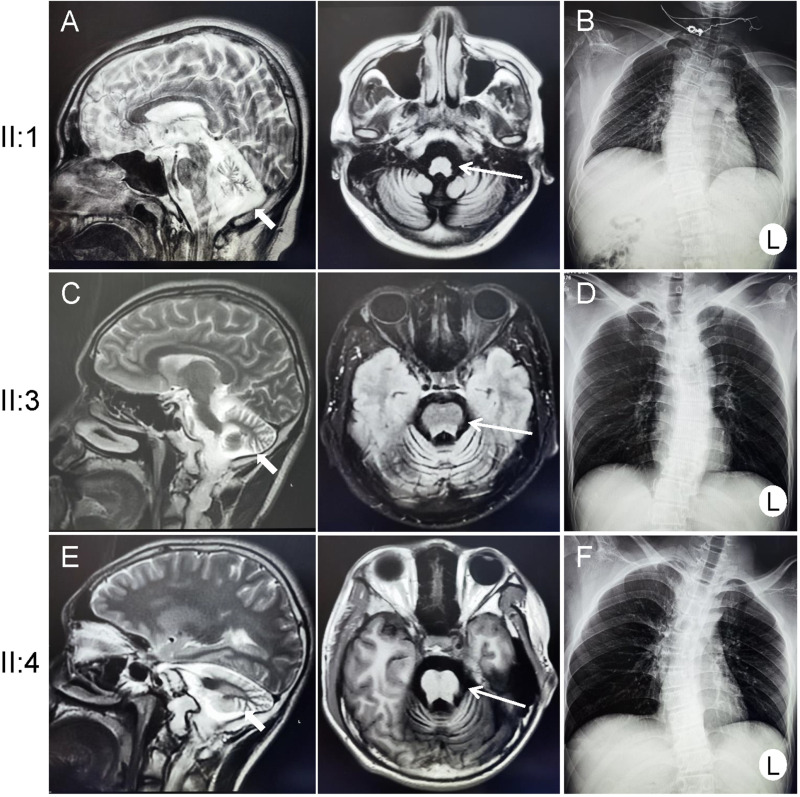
Radiological images of three patients (II:1, II:3, and II:4). Brain magnetic resonance imaging of three patients (A, C, and E) showing cerebellar atrophy (thick short arrow) and brain stem atrophy (thin long arrow), and chest X-ray showing scoliosis (B, D, and F).
